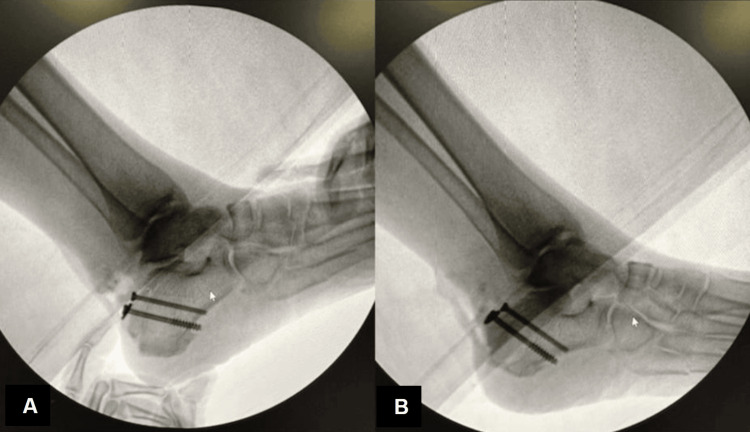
Final radiographs of reduced calcaneal fracture. Intraoperative and immediately postoperative lateral radiographs of the right foot displaying a reduced calcaneal fracture before and after Achilles tendon repair (A and B, respectively).
